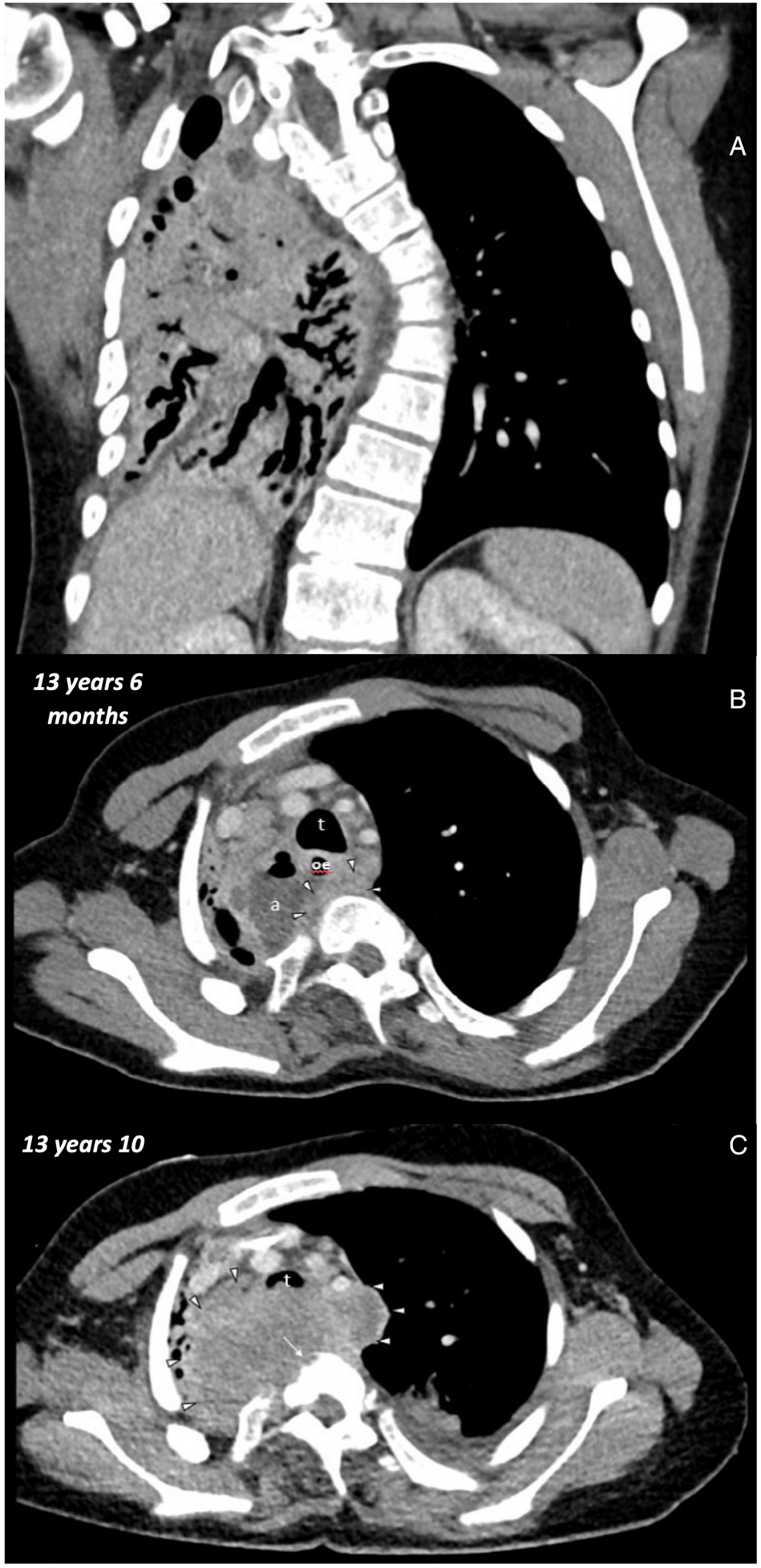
Initial chest CT at the age of 13 years 6 months: (A) coronal soft tissue window thorax: volume loss of the right lung with bronchiectasis. Left convex scoliosis. (B) Axial soft tissue window upper thorax: trachea (t), esophagus (oe), abscess (a), soft tissue mass (arrowheads). Second chest CT at the age of 13 Years 10 months: (C) axial soft tissue window upper thorax: trachea (t), soft tissue mass (arrows heads), bony errosions (long arrow).
